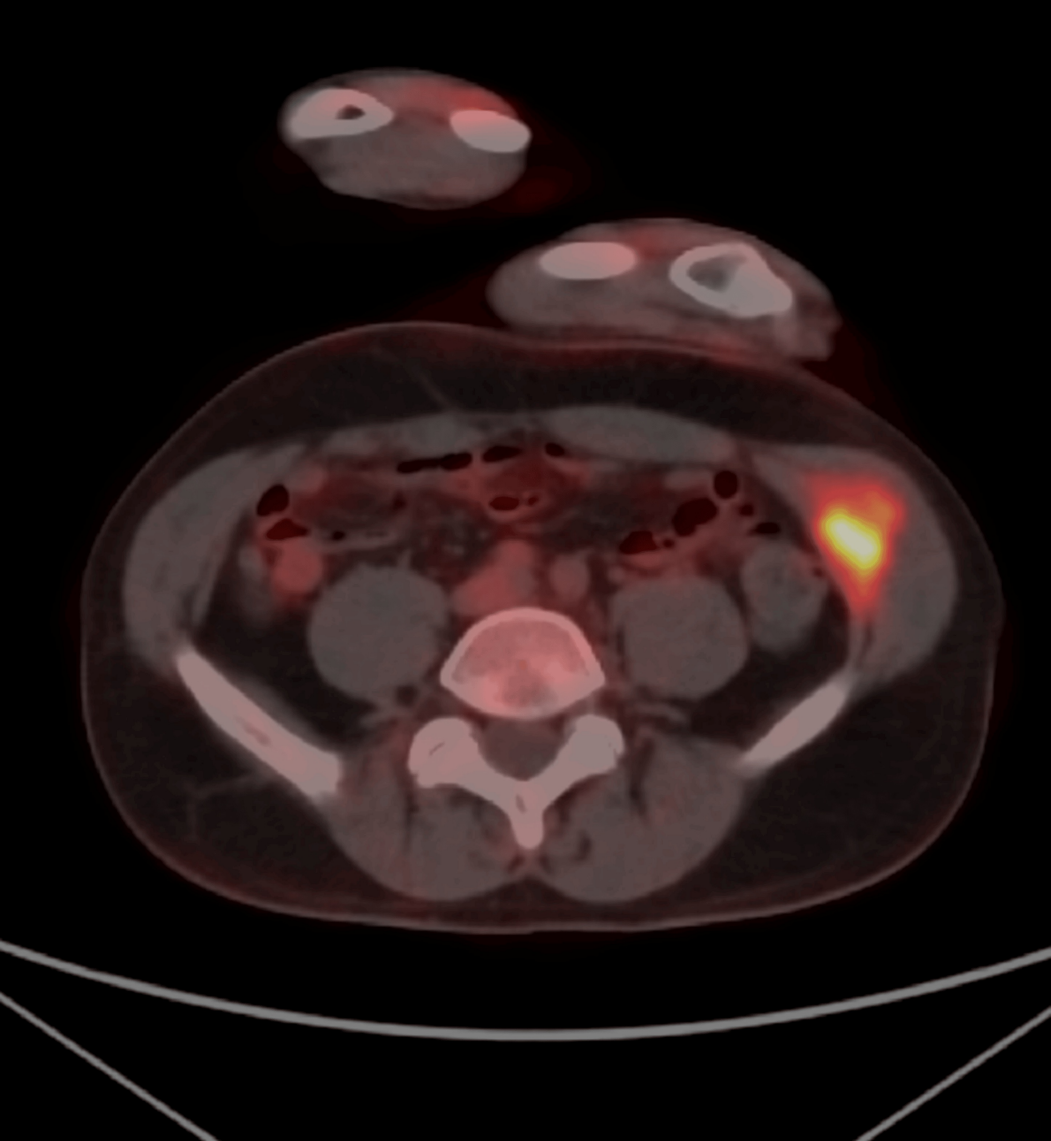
Positron emission tomography (PET)-computed tomography (CT) scan Asymmetry in size between the left and right internal oblique muscle of the abdominal wall due to a mass showing abnormal fluorodeoxyglucose (FDG) activity in the left.
